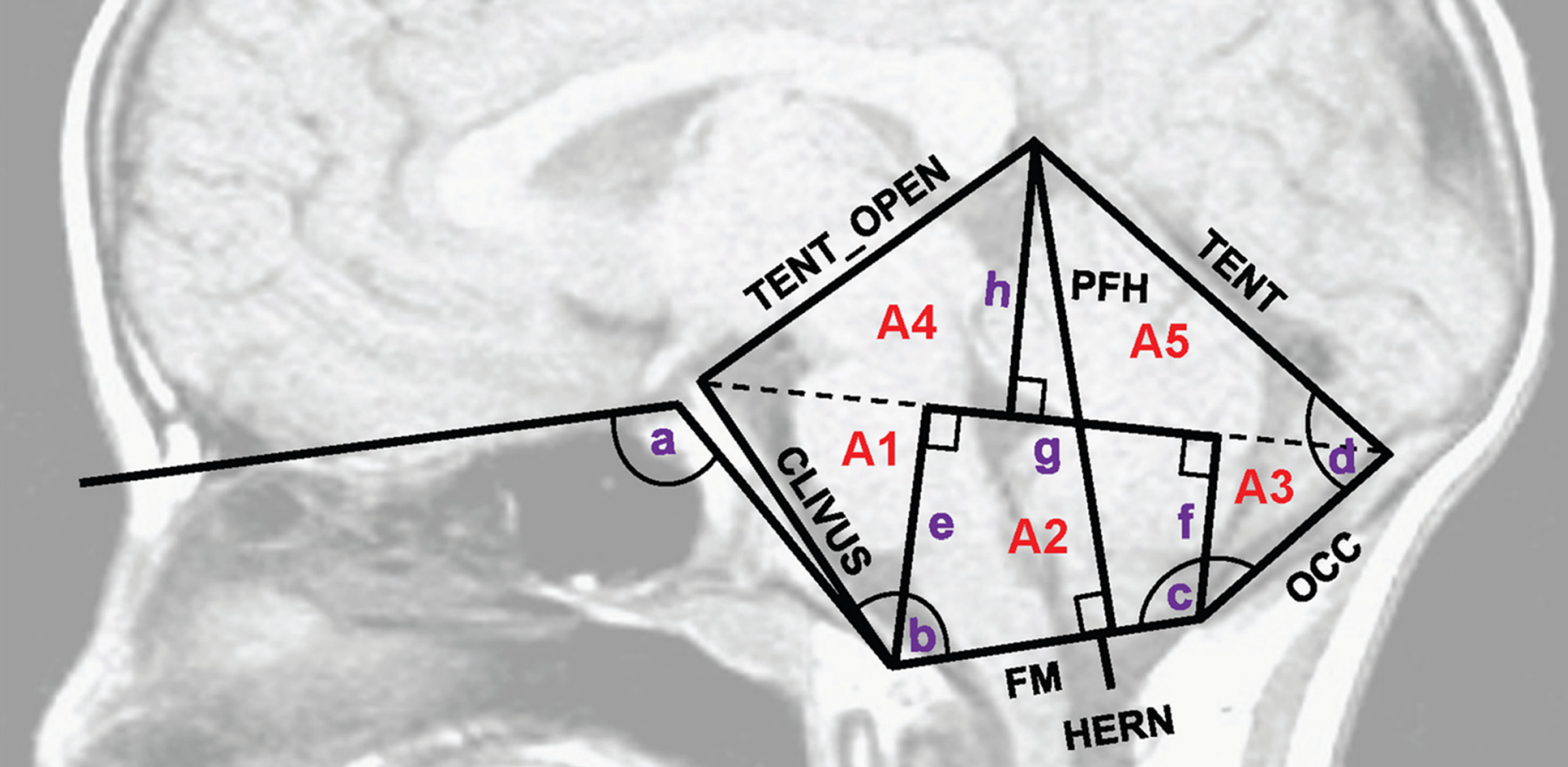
Posterior cranial fossa measurements taken from the midline of a sagittal, T1-weighted MRI. A1 to A5 shown in red indicate the regions in which area was estimated. Additional measurements not explicitly labeled include (a) basal angle (BASAL_ANG), (b) boogaard angle (BOOG_ANG), (c) occipital angle (OCC_ANG), (d) tentorial angle (TENT_ANG), (e) basion to reference (BASTOREF), (f) opisthion to reference (OPISTOREF), (g) trapezoid height (TRAPHEIGHT), and (h) tentorium to reference (TENTTOREF). Abbreviations: TENT_OPEN = tentorial opening, TENT = tentorium, OCC = supraoccipital bone, FM = foramen magnum, PFH = posterior fossa height, and HERN = cerebellar tonsillar herniation. This figure is reproduced from
